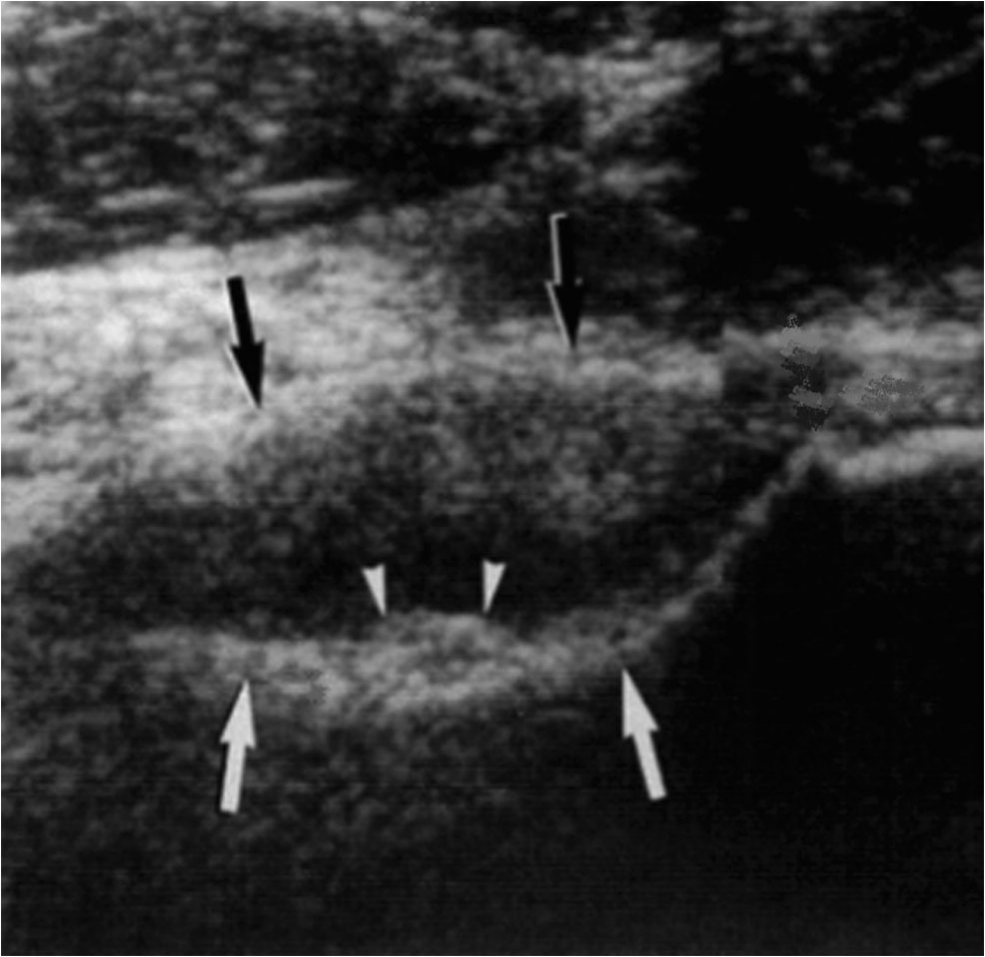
An ultrasound image of the hip shows synovial/capsular swelling (black arrows) as well as a small nodule with an echogenic surface (arrowheads) on the anterior aspect of the femoral neck (white arrows). This small nodule corresponded to a very superficial OO identified on CT (not shown) (from Malghem J et al. [57])
